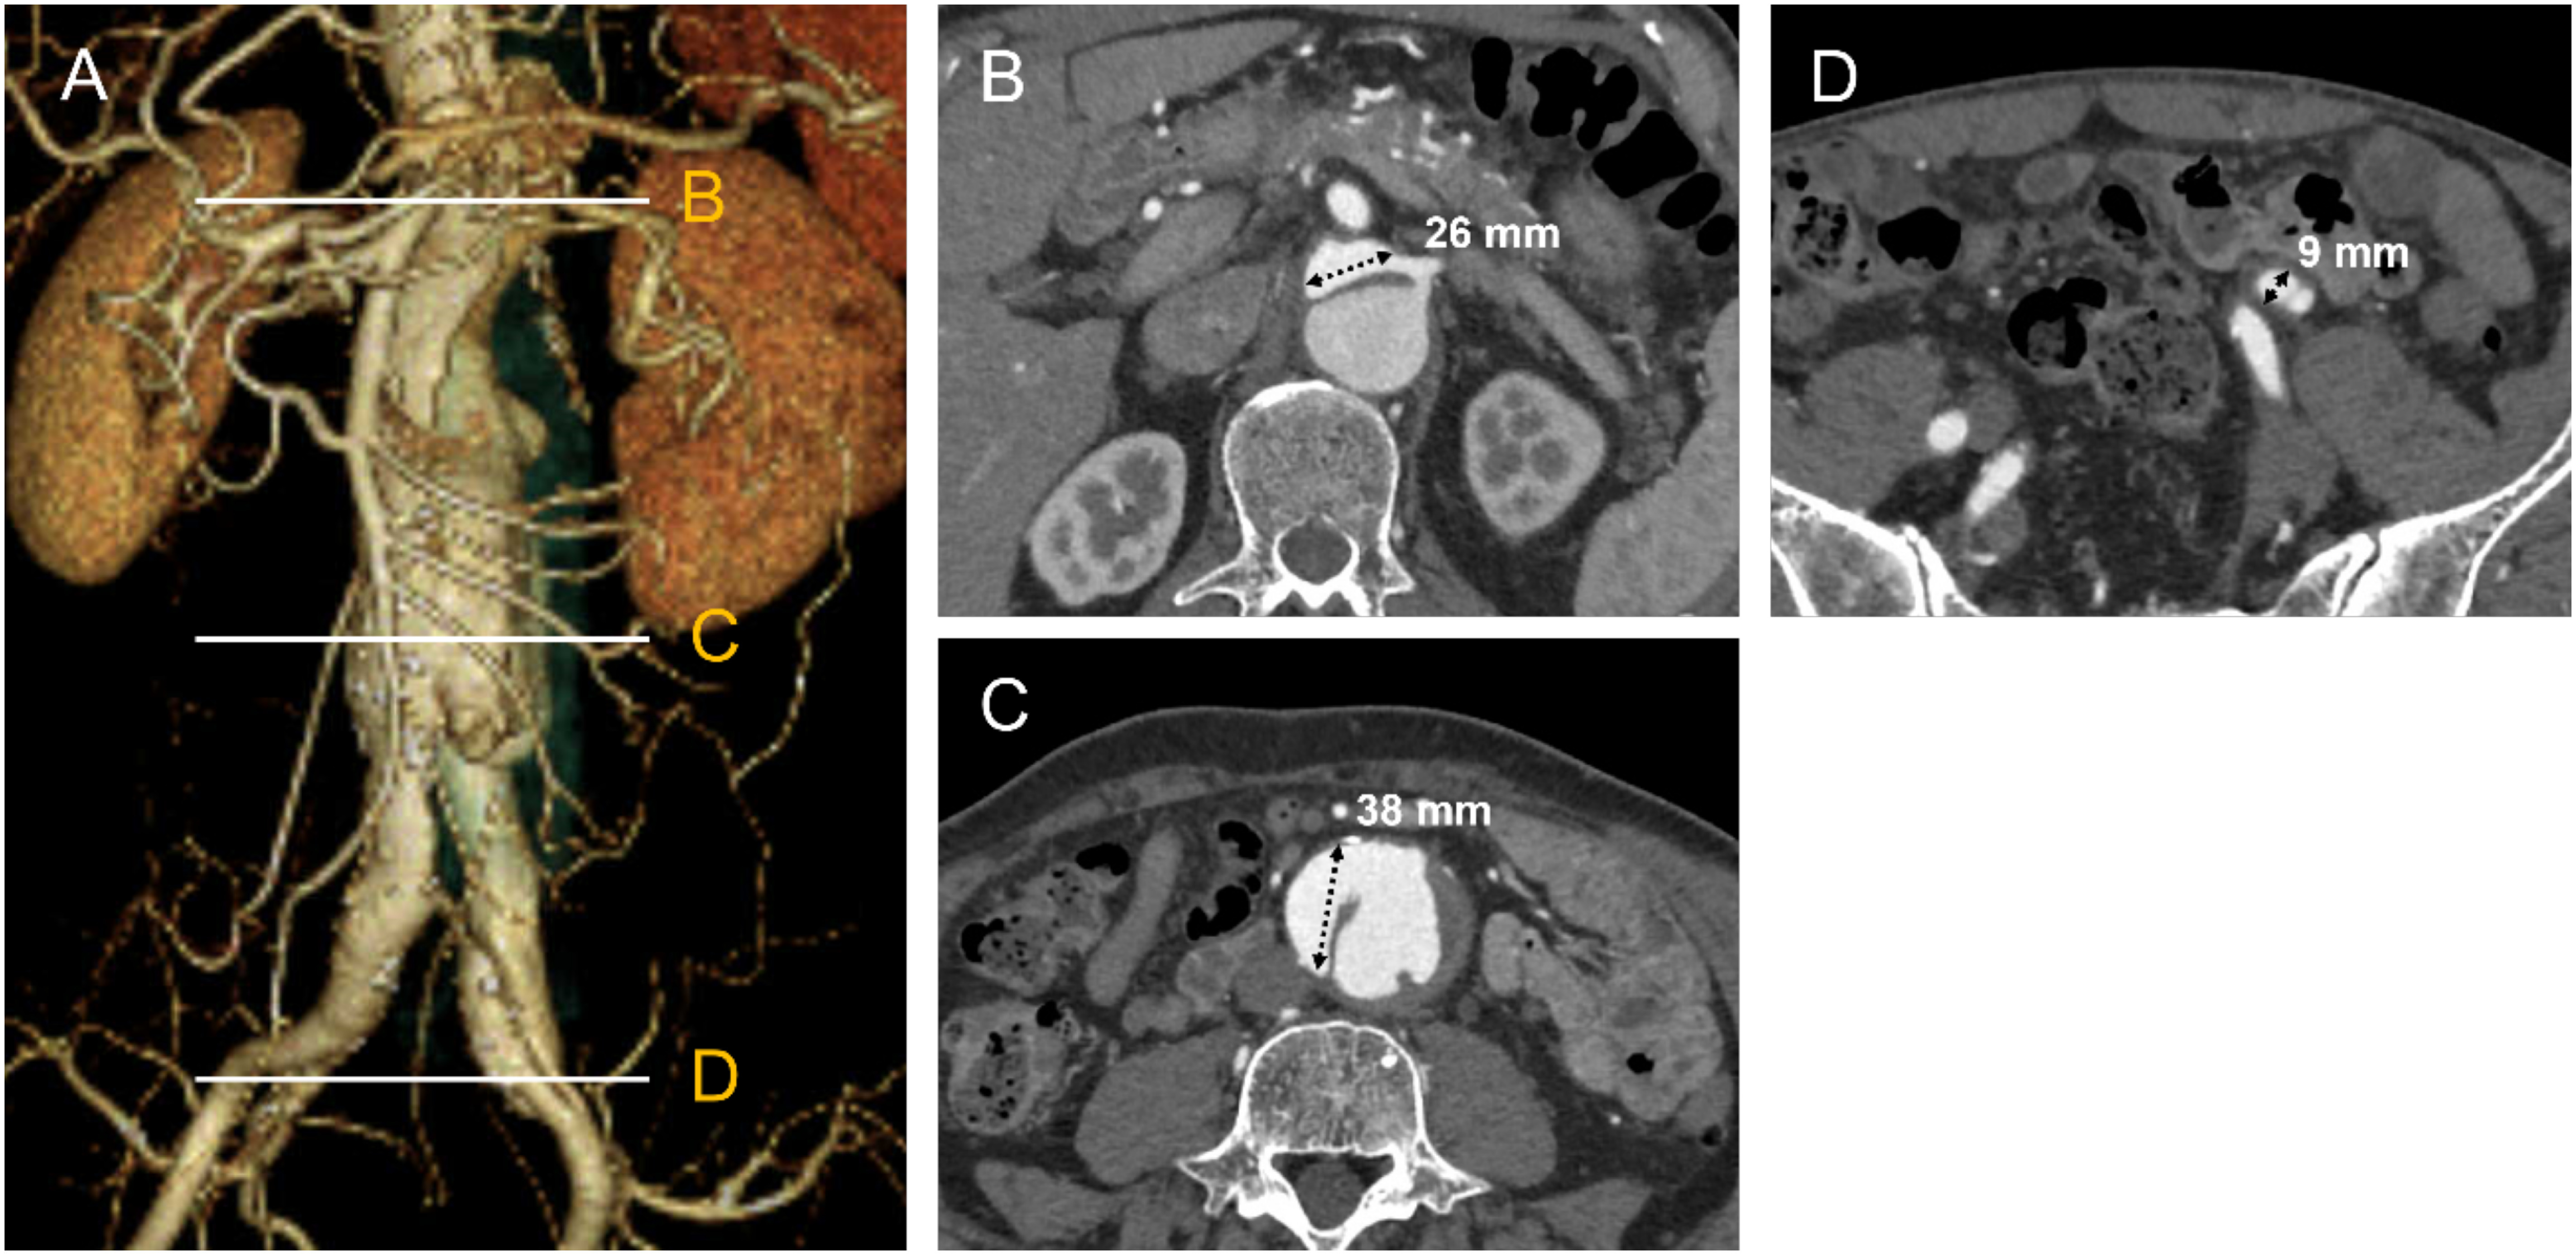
Fig. 1 Preoperative volume rendering showing the false lumen flow from the proximal abdominal aorta to the left external iliac artery (EIA) (A). Contrast-enhanced computed tomography axial images in the early phase showing the entries at the left renal artery orifice (B), descending aorta (C), and the left EIA (D).
